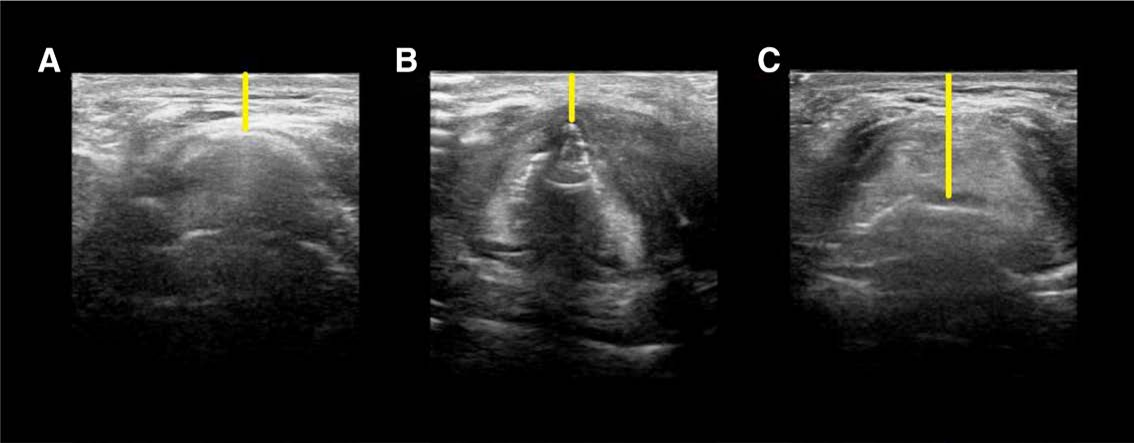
Ultrasound images of the measurements. Ultrasound distances: (A) DSHB, distance between the hyoid bone and skin; (B) DSAC, distance between the vocal cord anterior commissure and skin; (C) DSE, distance between the epiglottis and skin.
